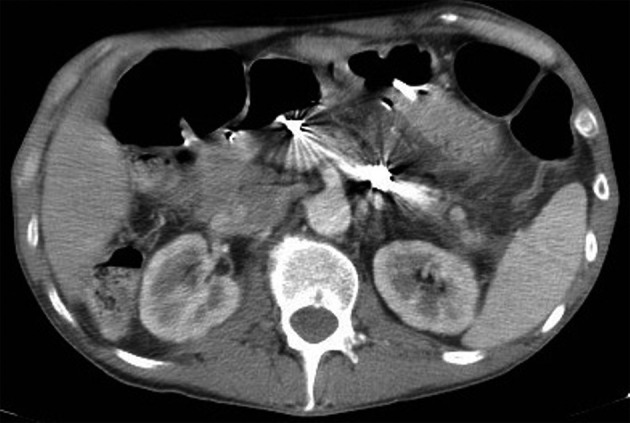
CT scan performed 5 months following EUS guided arterial pseudoaneurysm embolization.
